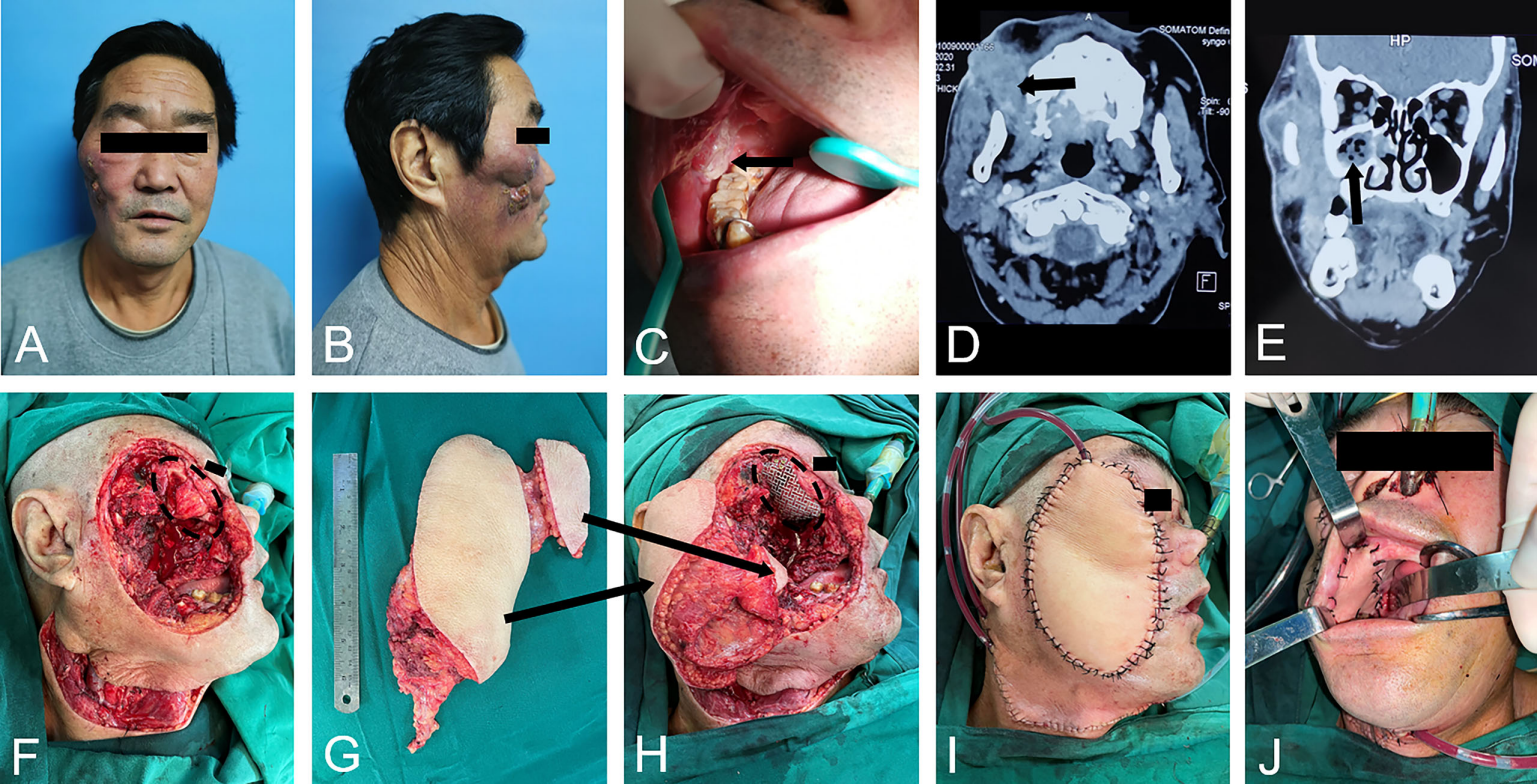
A typical salvage surgery case. (A-C). primary tumor outside and inside the mouth; (D, E). radiographic images presented tumor encroached maxillary and mandibular bone, maxillary sinus and orbital floor; (F). excision of tumor led to orbit exposure; (G, H). reconstruction with double-skin paddle free latissimus dorsi flaps and Titanium mesh implantation of right orbit; (I, J). immediate postoperative images.
